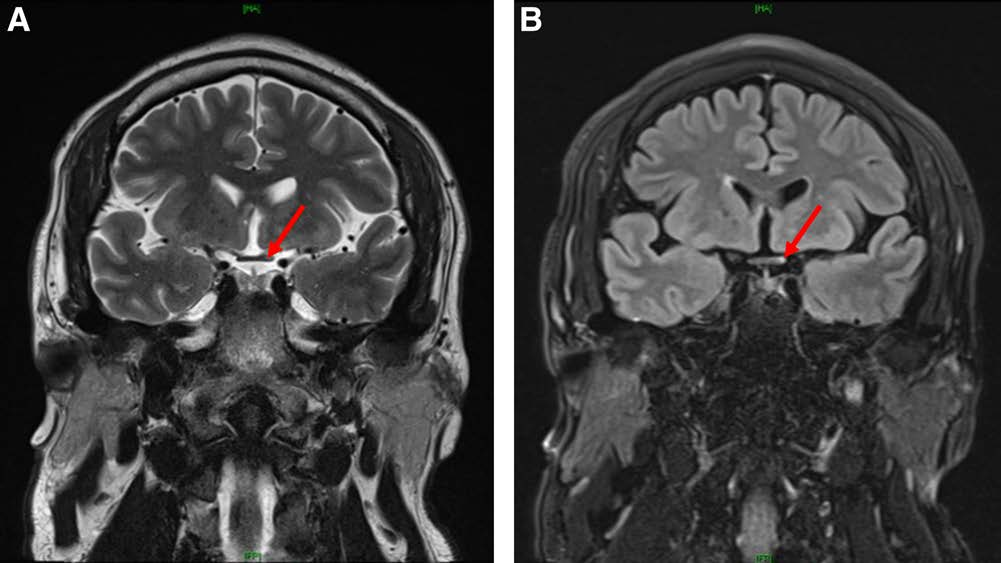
Brain magnetic resonance imaging of the left optic chiasm and the prechiasmatic optic nerve segment. (A) T2 weighted coronal image, (B) Fluid attenuated inversion recovery (FLAIR) image. Red arrows showed swelling with hyperintensity of the left optic chiasm and prechiasmatic optic nerve segment.
